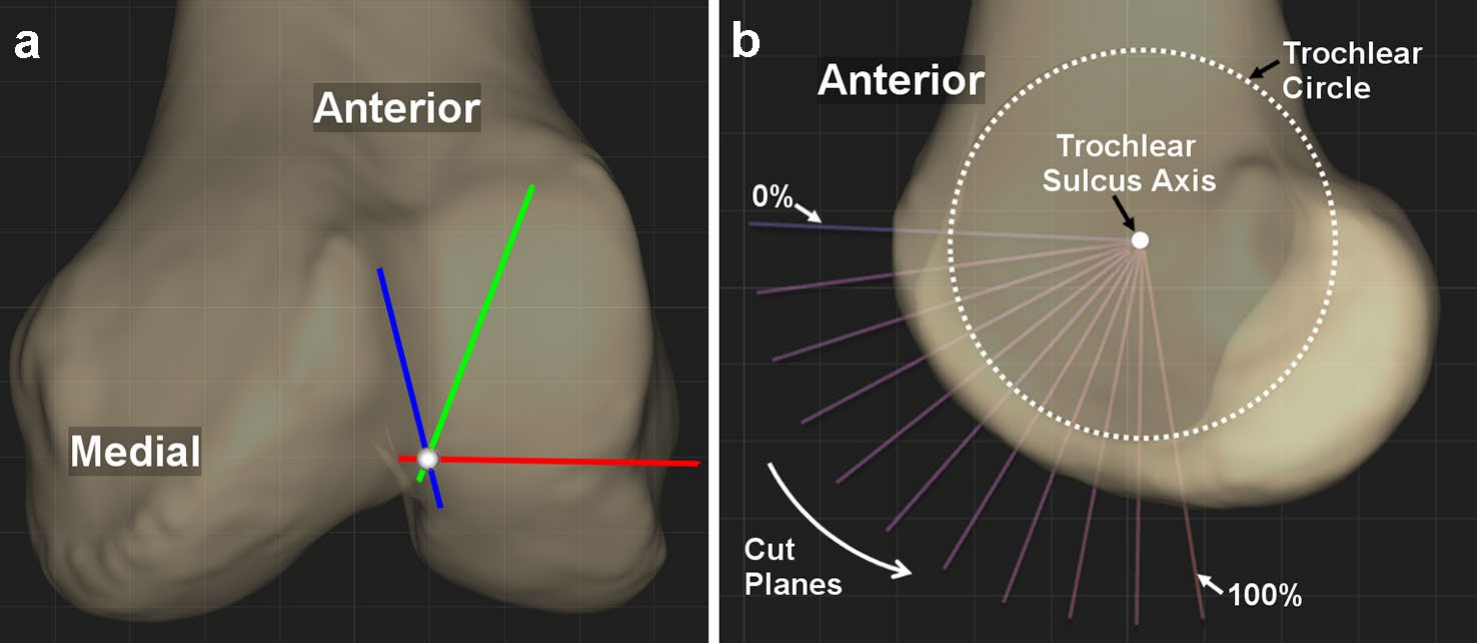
Constructions on the left femur of a representative CT bone model. A Coordinate system established on each femur, with + Z (blue) pointing superior along the mechanical axis, + Y (green) pointing anterior perpendicular to the surgical transepicondylar axis, + X (red) pointing lateral, centred at the apex of the intercondylar notch. B The trochlear sulcus axis was established as the line crossing the centre of this circle and parallel to the cylindrical axis. A total of eleven coaxial cutting planes rotated equally about the trochlear sulcus axis were created. Each coaxial cutting plane was defined by the percentage along the arc of the trochlear sulcus with 0% and 100% intersecting the most anteroproximal and posterodistal points, respectively
